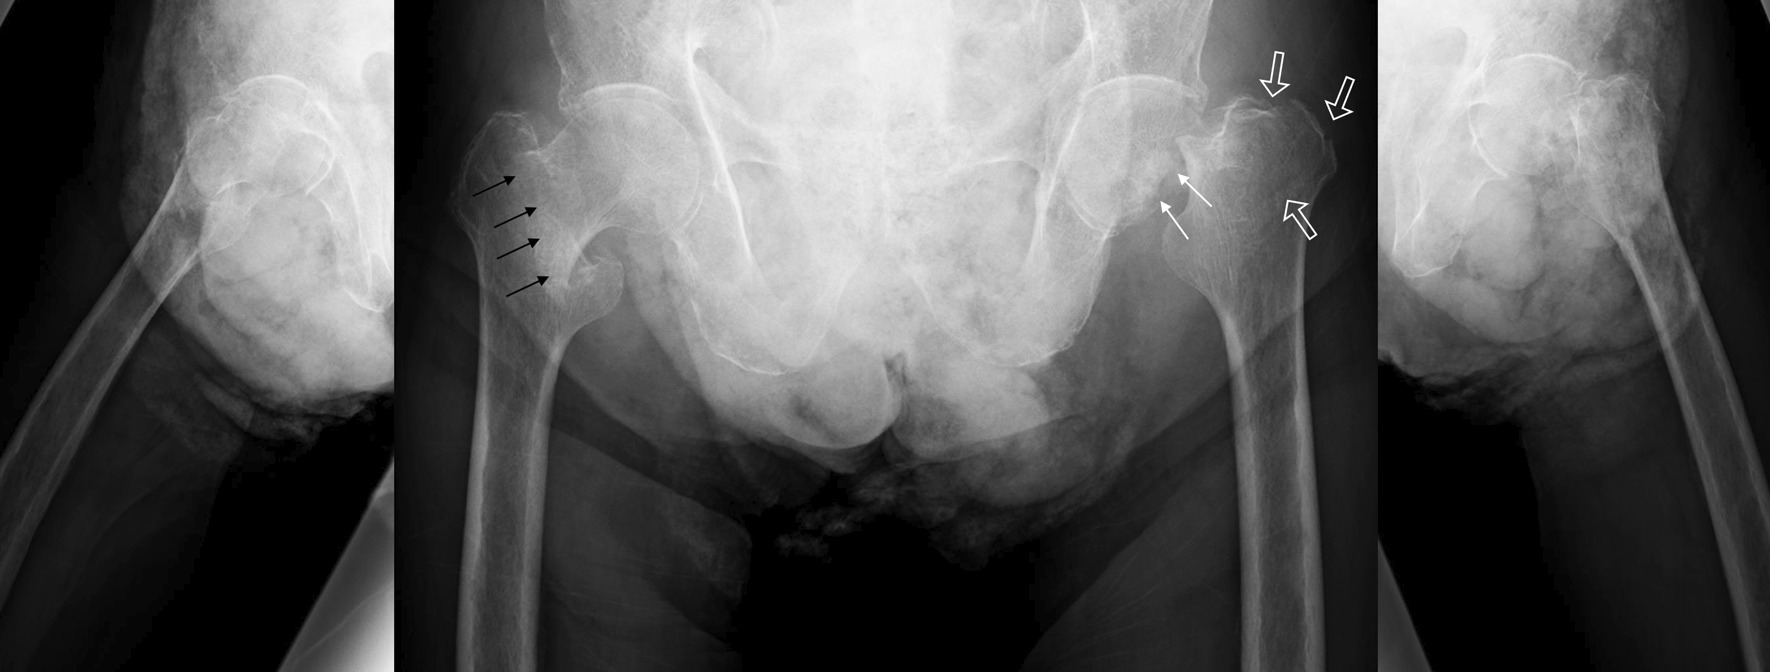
Radiography findings, showing left femoral neck fracture (white arrow), left greater trochanteric fracture (white outline arrow), and right femoral trochanteric fracture (black arrow)
